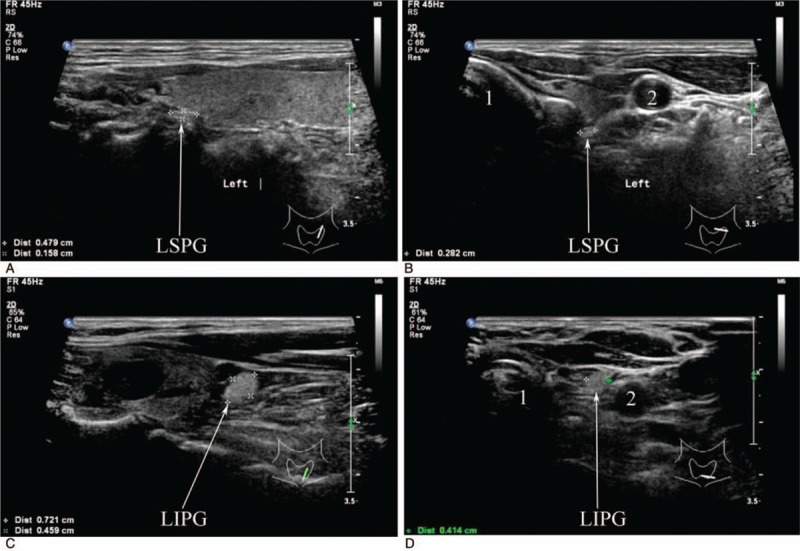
Transverse location of the normal parathyroid glands. (A) (longitudinal scan) and (B) (transverse scan), ultrasonographic appearance of a LSPG located on the dorsal part of the upper third thyroid lobe and in proximity to the trachea; (C) (longitudinal scan) and (D) (transverse scan), ultrasonographic appearance of a LIPG placed below the lower thyroid lobe and close to the common carotid artery. LIPG = left inferior parathyroid gland; LSPG = left superior parathyroid gland.
